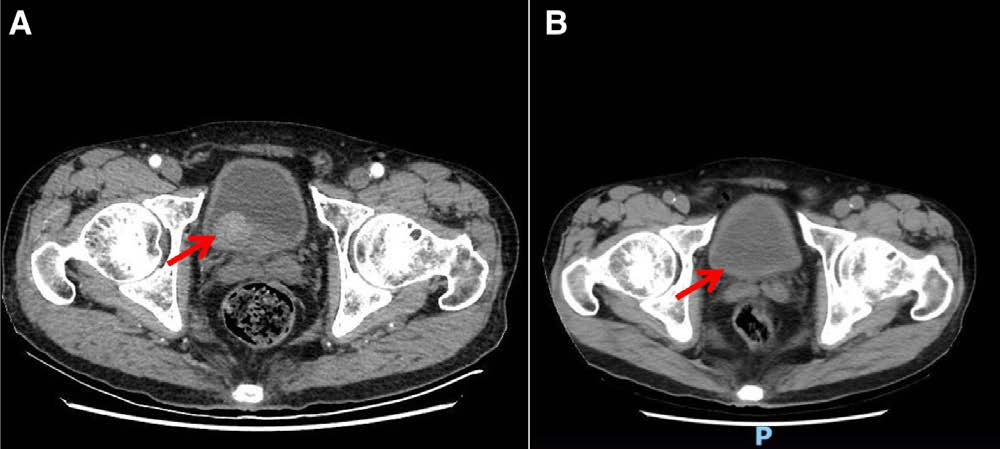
Images of the patient throughout the treatment. (A) Before treatment, abdomen CTU showed localized invasion of the right wall lump of the bladder into the surrounding adipose tissue. (B) After maximal TURBT combined with tislelizumab, abnormal signals was seen on abdomen enhanced CT. CTU = computed tomography urography, TURBT = transurethral resection of the bladder tumor.
